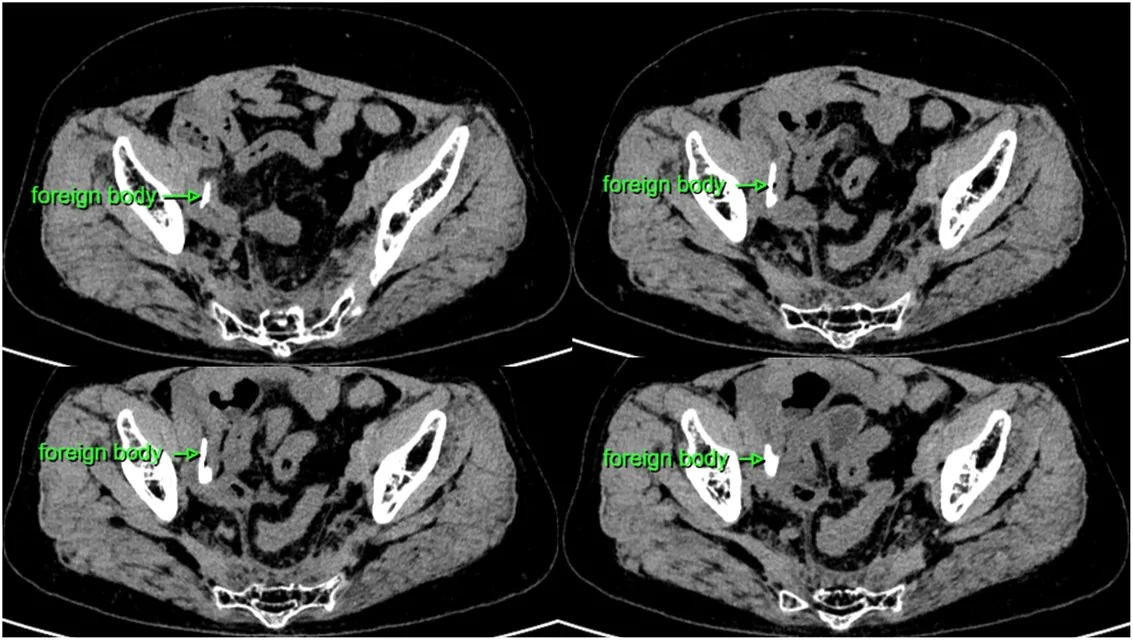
Preoperative abdominal CT (plain scan) obtained prior to open surgical removal of the foreign body shows a strip-like hyperdense foreign body (arrow) in the right pelvic small intestine, with adjacent mesenteric haziness indicating inflammatory changes.
